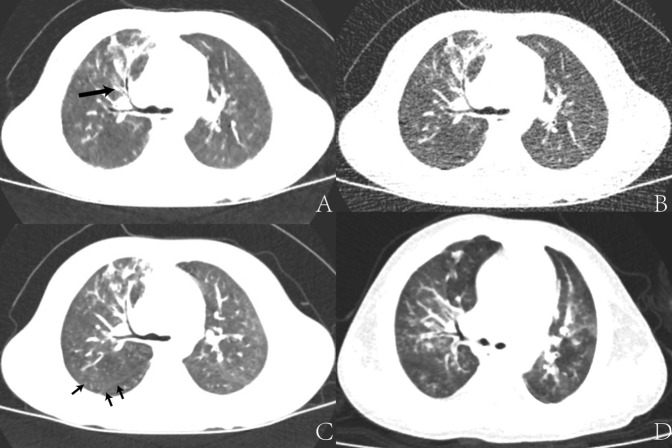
Images of lung window acquired at different dose levels and with different reconstruction algorithms. (A–C), 3 years old boy with pneumonia. (A) Low dose images, radiation dose was 0.08 mSv, reconstructed with MBIR (LD-MBIR). (B) Low dose images, reconstructed with ASIR50% (LD-ASIR). (C) Routine dose CECT images, radiation dose was 1.34 mSv, reconstructed with ASIR50%. The noise of the LD-MBIR images was similar to that of the Routine dose CECT ASIR images. However, LD-MBIR images were inferior to CECT ASIR images in displaying fine lung markings and pleural shadows (short arrow). The bronchial lumen with LD-MBIR (long arrow) was clearer than LD-ASIR. (D) Another 3 years old girl with pneumonia. Routine dose non enhanced CT images, radiation dose was 1.43 mSv, reconstructed with ASIR50% (RD-ASIR). The bronchial lumen was similar to LD-MBIR, but the performance of lung markings was better than LD-MBIR.
